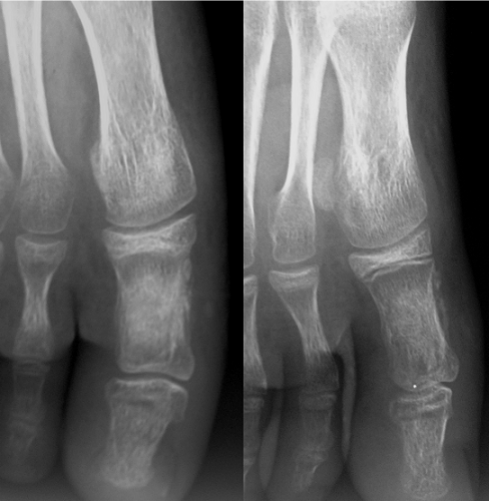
Anteroposterior and sagittal radiographs 6 mouths postoperatively: bone satisfying appearance and incorporated bone graft
